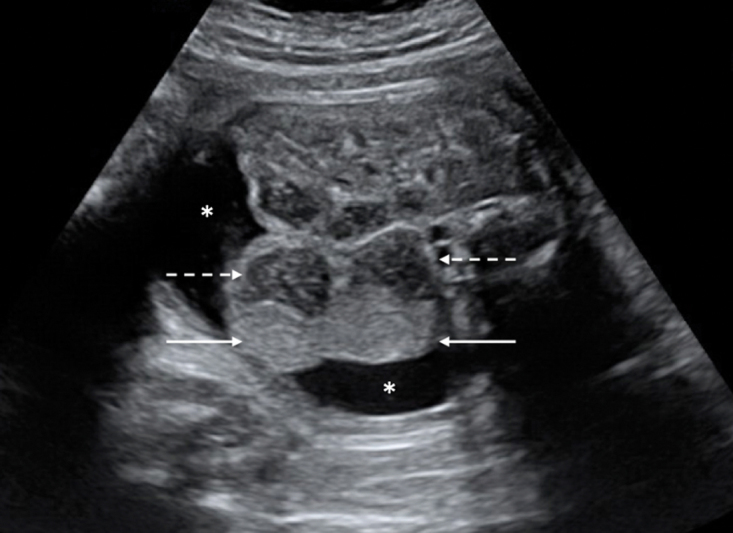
Prenatal ultrasound, transverse slice at the level just below the liver: visualization of a hyperechogenic mass (white solid arrows) adjacent to a dilated digestive loop (white dotted arrows), surrounded by anechogenic ascites (asterisk [*]) in the right hemiabdomen.
